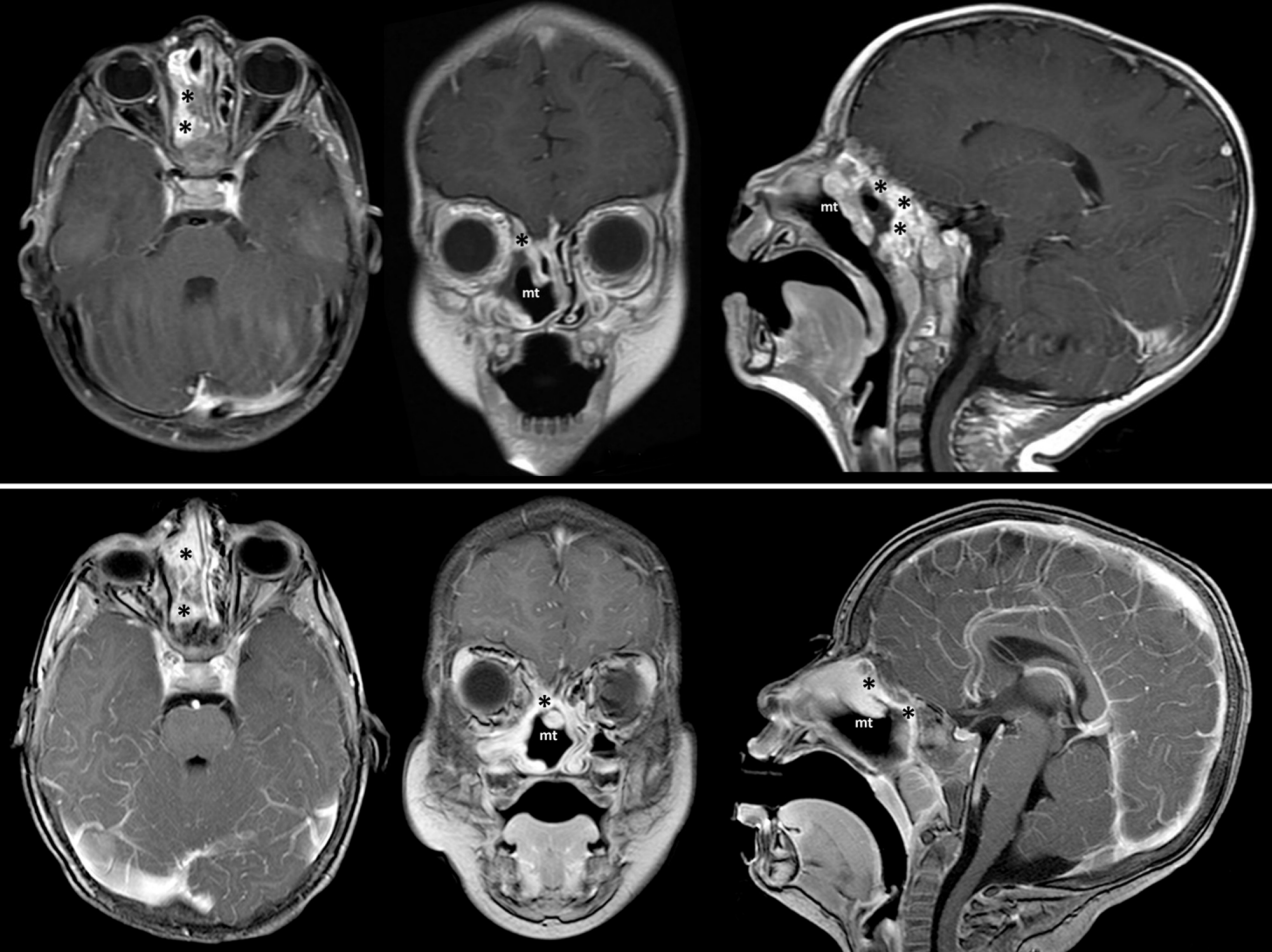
Follow-up MRI. Top: at six months of follow-up after the second surgery. Bottom: at 12 months after the second surgery Lateral nasal wall is completely free of tumor mass; however, the remnants are visible along the ethmoid roof and in the sphenoid sinus (asterisks). There is no evidence of tumor progression. Decrease of inflammatory changes is observed. (mt – middle turbinate)
